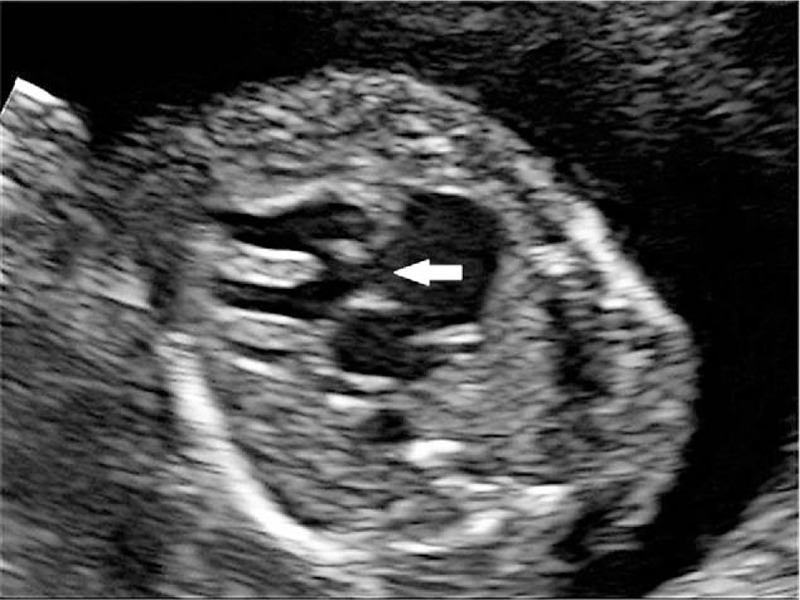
The sonographic examination of case 1 at 21 weeks’ gestational age. Four-chamber view of the fetal heart showed atrioventricular septal defect (white arrowhead).
